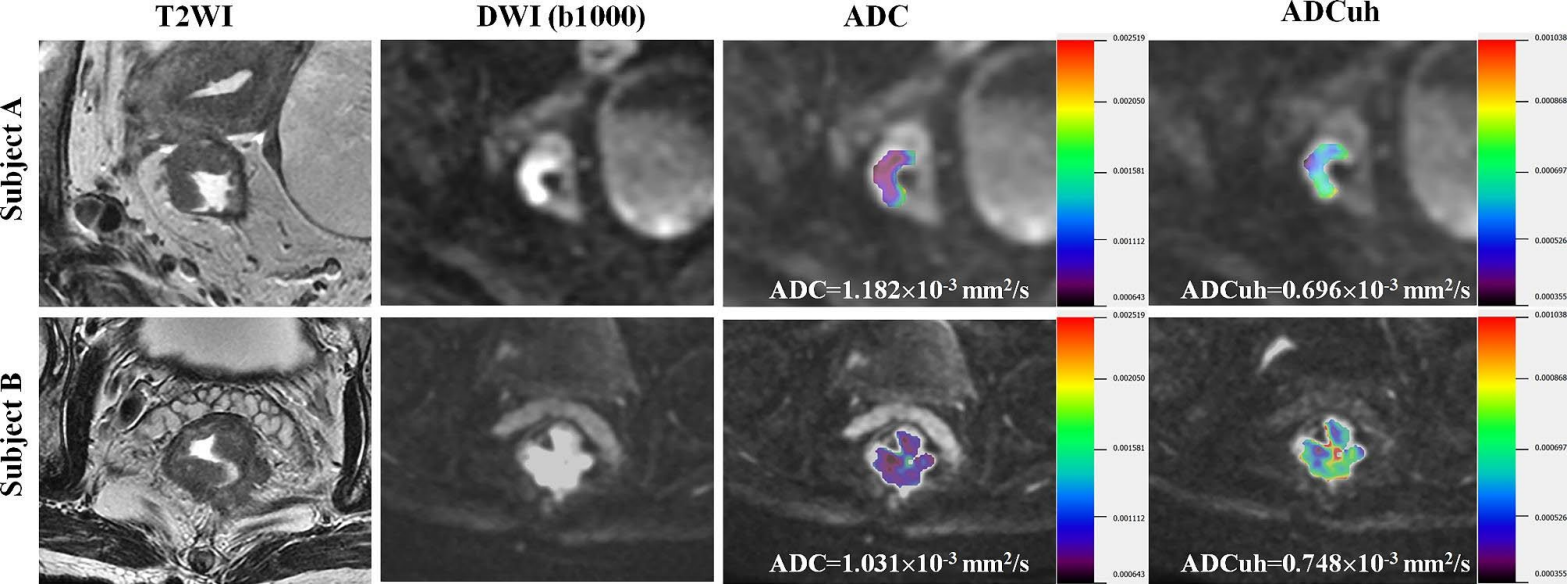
MR images of patients. Subject A (female, 76-year-old, TNM-stage II, EMVI- and MRF-) had no progression during follow-up. According to the cutoff values of ADC (> 1.140 × 10^− 3^ mm^2^/s) and ADCuh (> 0.716 × 10^− 3^ mm^2^/s), wrong prediction about progression for subject A will be made based on ADC value, while right prediction will be given based on ADCuh value. Subject B (male, 57-year-old, TNM-stage III, EMVI+, MRF+) had multiple organ distant metastases and died during follow-up. Wrong prediction for subject B will be made based on ADC value, in contrast, accurate prediction will be given based on ADCuh value
